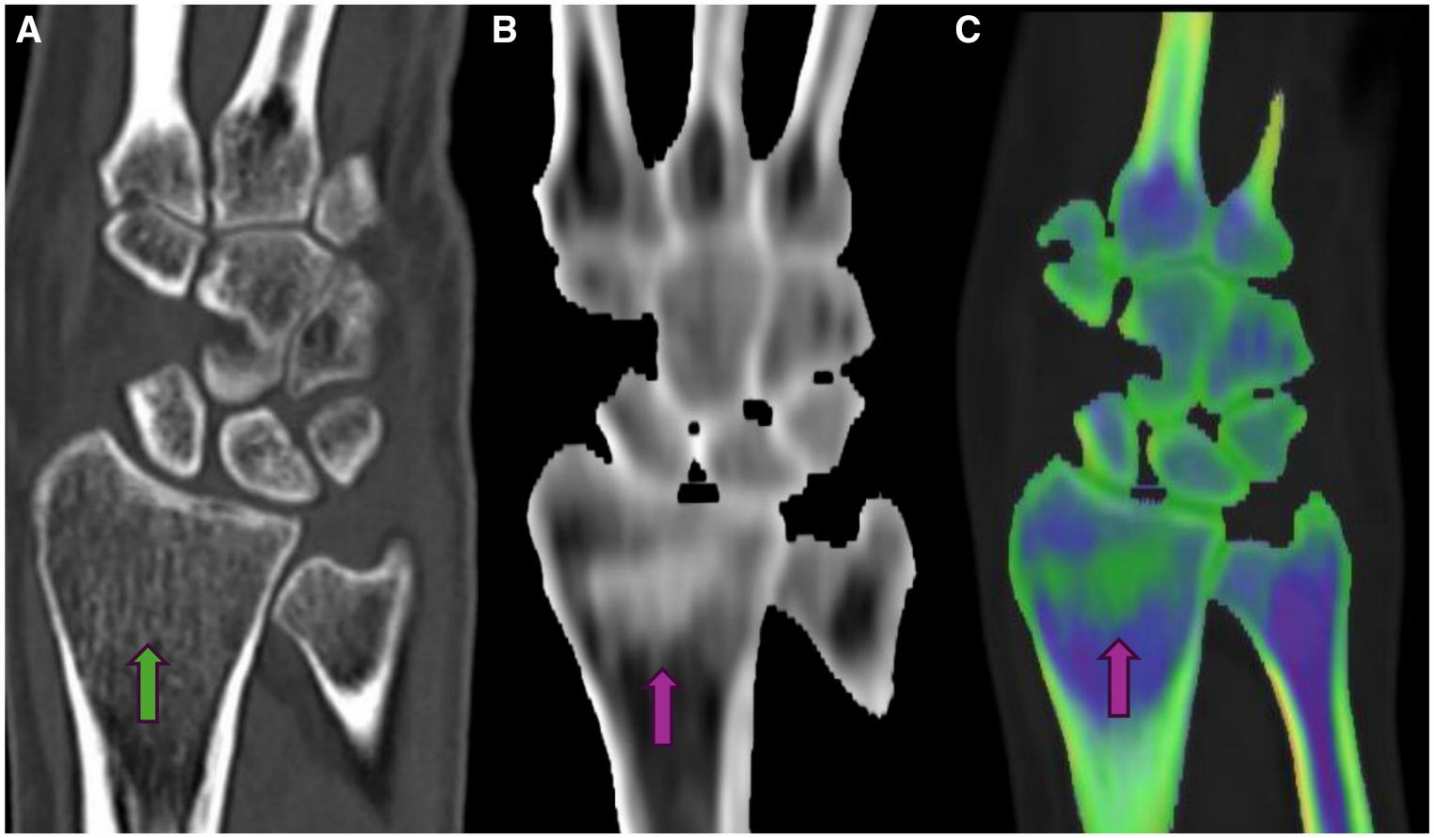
Patient with a history of acute wrist injury. A (green arrow): routine CT showing nonfracture or oedema. B and C show marrow oedema on DECT suggestive of a micro-trabecular fracture.
